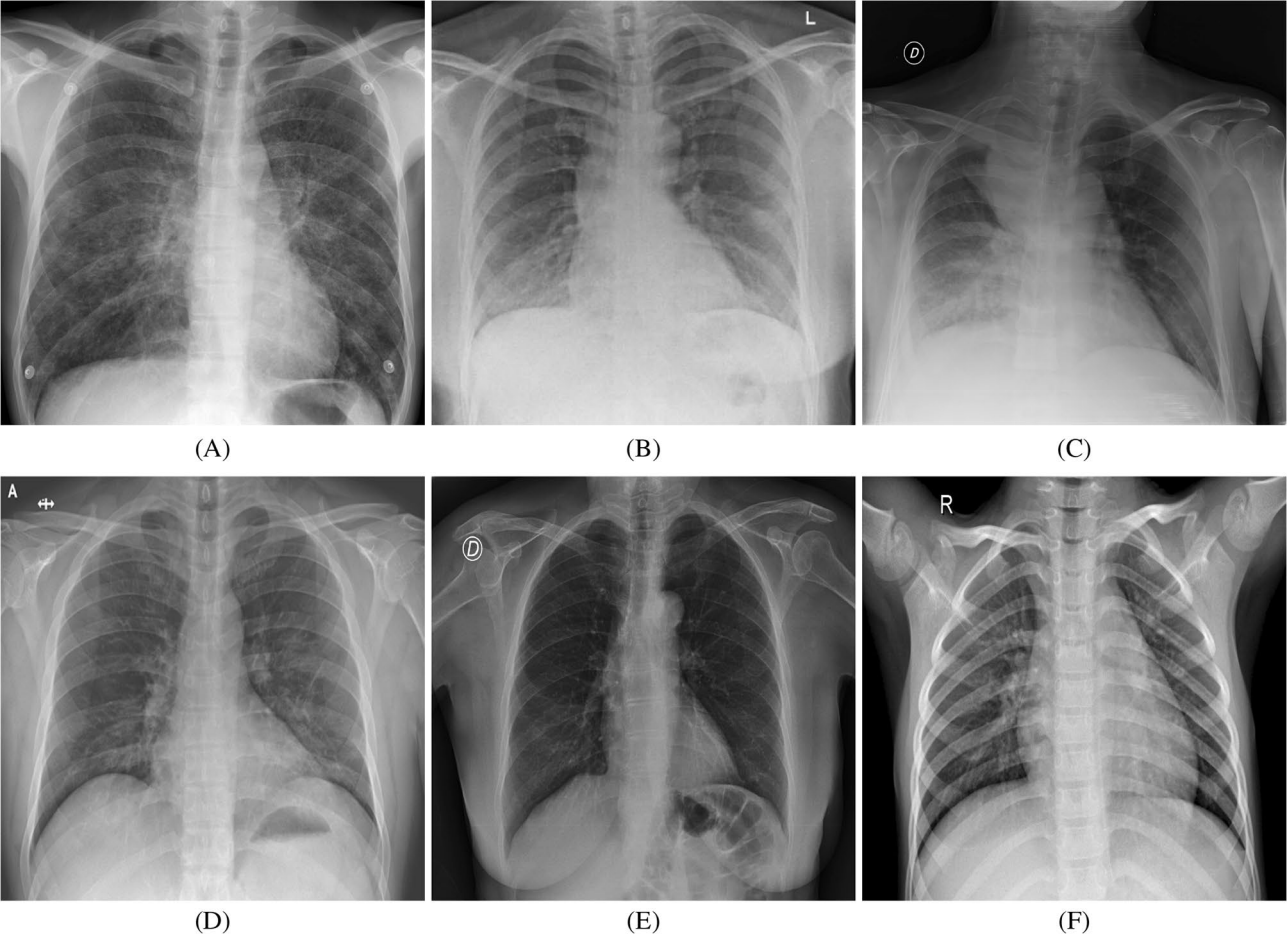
Examples of X-ray chest radiography images collected from COVID-19 patient and normal cases. (A) A COVID-19 patient case from COVID-19 open image data collection. (B) A COVID-19 patient case from Fig. 1 COVID-19 Chest X-ray Dataset Initiative. (C) A COVID-19 patient case from ActualMed COVID-19 chest X-ray dataset initiative. (D) A COVID-19 patient case from COVID-19 Radiography Database. (E) A normal case from ActualMed COVID-19 Chest X-ray dataset initiative. (F) A normal case from COVID-19 radiography database.
